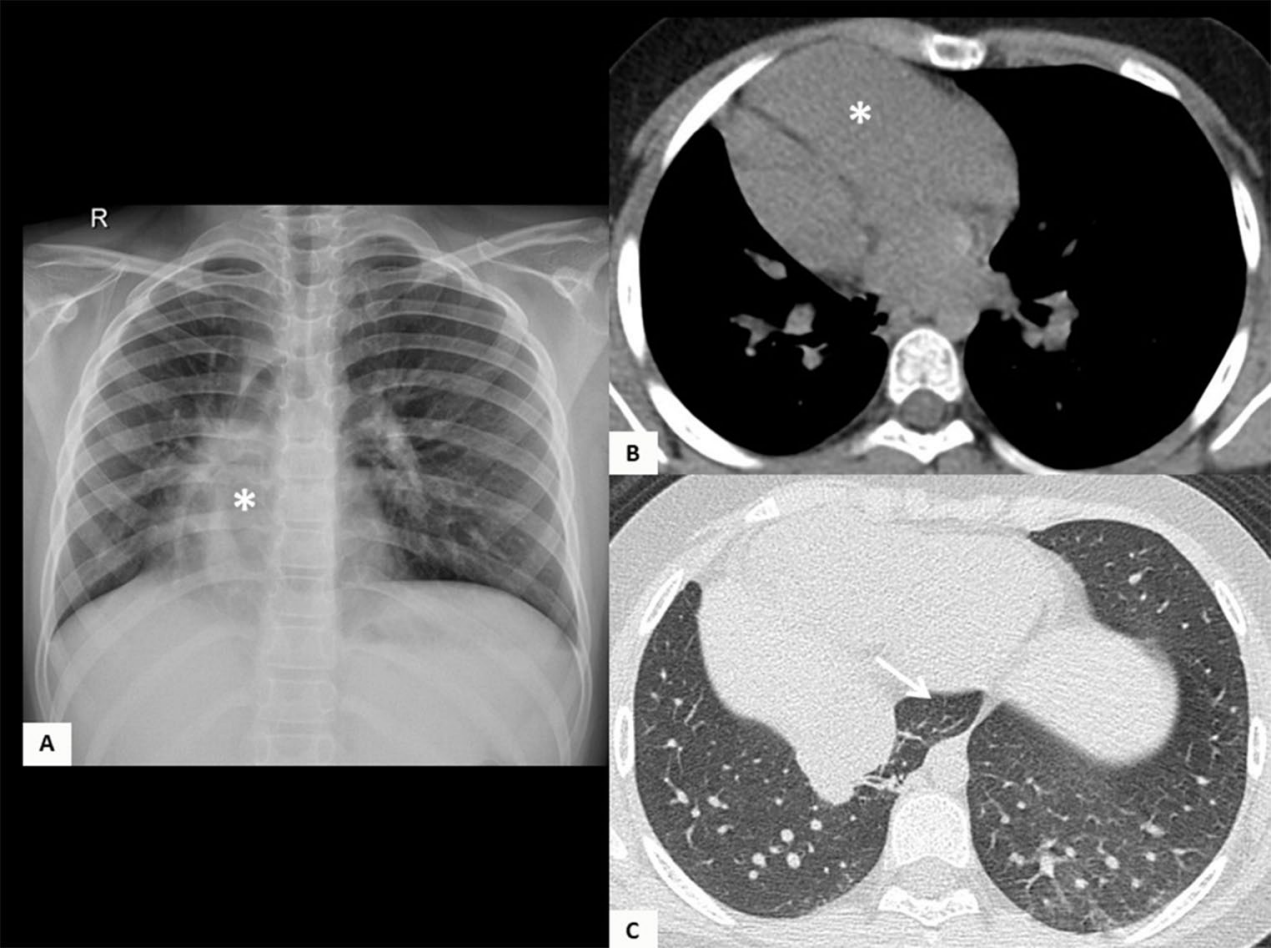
4-year-old female patient who had fallen a height, where findings were found incidentally. (A) Chest X-ray identified dextrocardia (asterisk). This was confirmed with a (B-C) chest CT scan revealing horseshoe lung (arrow in C)
